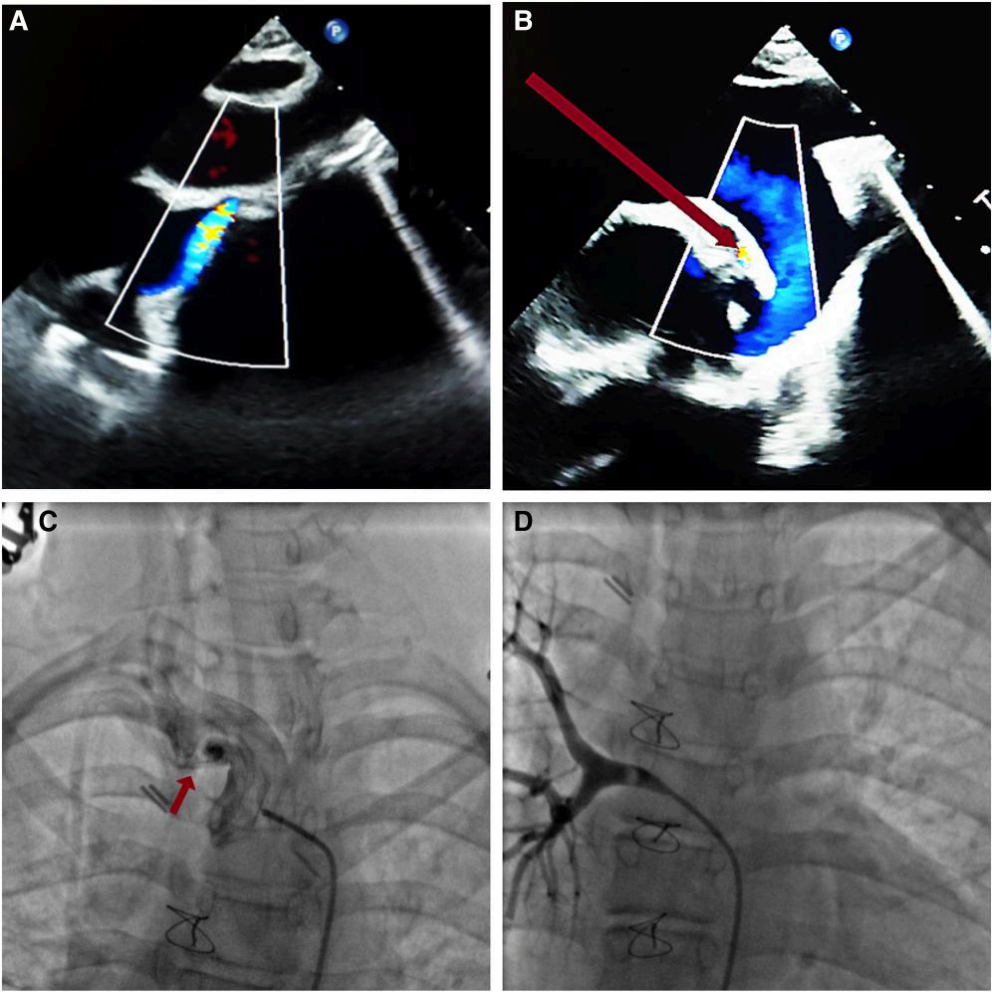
(*A* and *B*) Suprasternal view in transthoracic echocardiography showing aliasing in the MBTS (*A*) and major aorto-pulmonary collateral artery (*B*, arrow). (*C and D*) Thoracic angiography showing obstructed MBTS (*C*) and right MAPCA (*D*, arrow).
